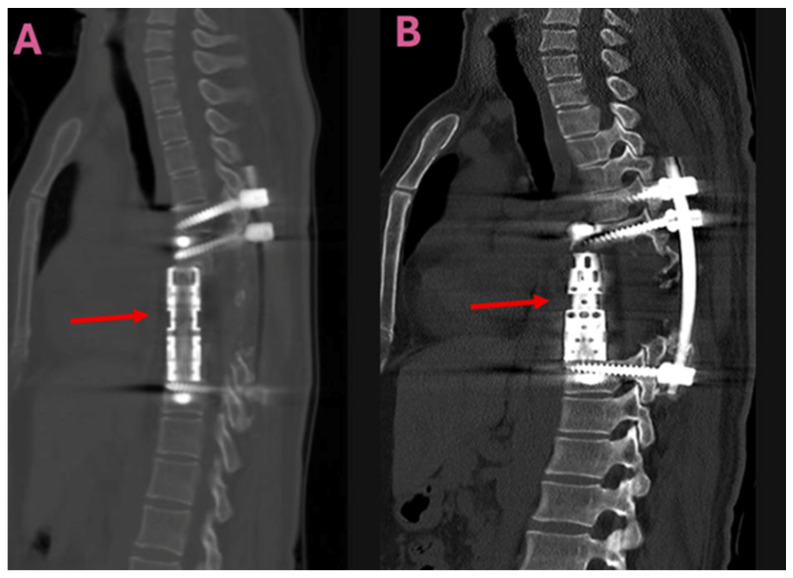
Postoperative sagittal CT images taken following total en-bloc spondylectomy (TES) for a thoracic spinal hydatid cyst. (A,B): Sagittal CT scans demonstrating vertebral body reconstruction and posterior instrumentation. Arrows indicate the titanium interbody cage used for vertebral body reconstruction. Abbreviations: CT, computed tomography; TES, total en-bloc spondylectomy.
